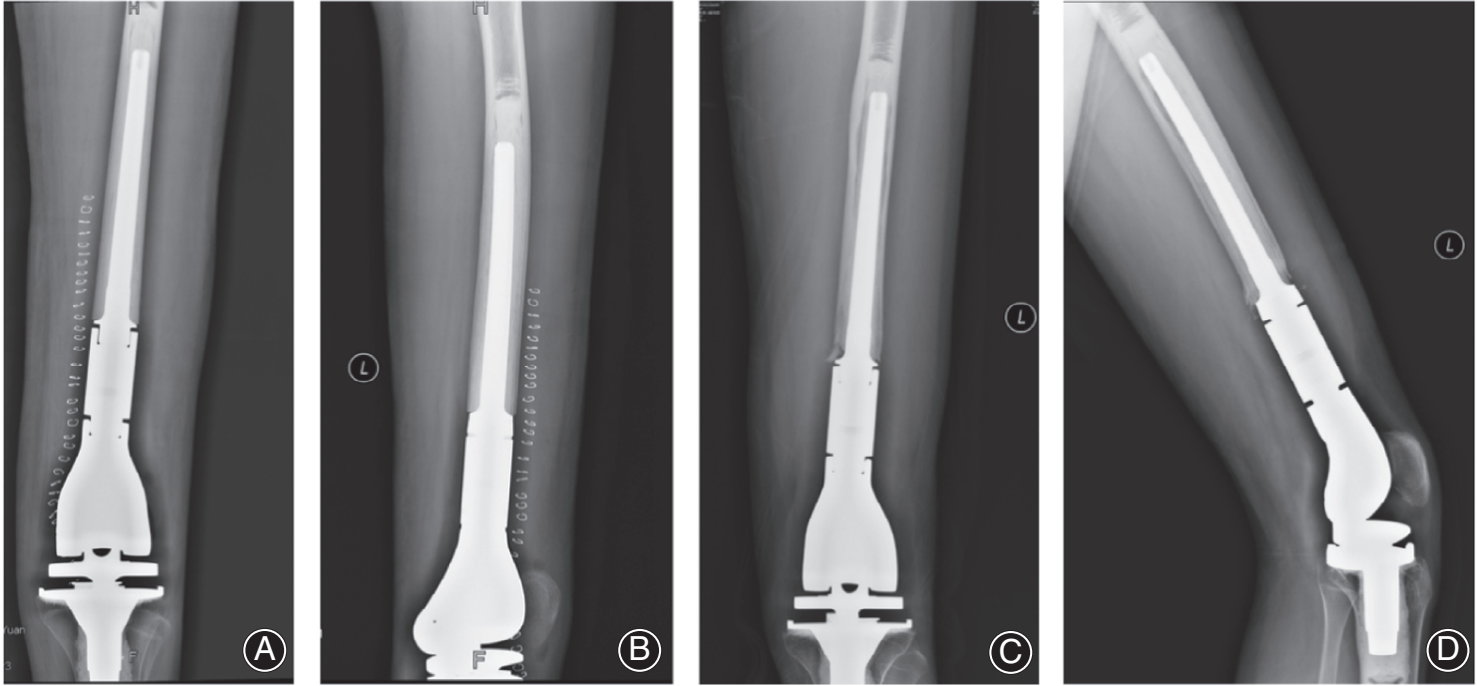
Aseptic loosening of the distal femoral component in a 14‐year‐old male treated for an osteosarcoma using cemented fixation of the distal prosthesis. Frontal (A) and lateral (B) views of the distal femoral component after surgery show a centralized positioning of the stem, with a translucent band visible between the cement layer of the proximal prosthesis stem and the cortical bone. Frontal (C) and lateral (D) view of the distal femur obtained 37 months after the surgery, with the translucent band being larger than on the initial baseline radiographs obtained post‐surgery.
